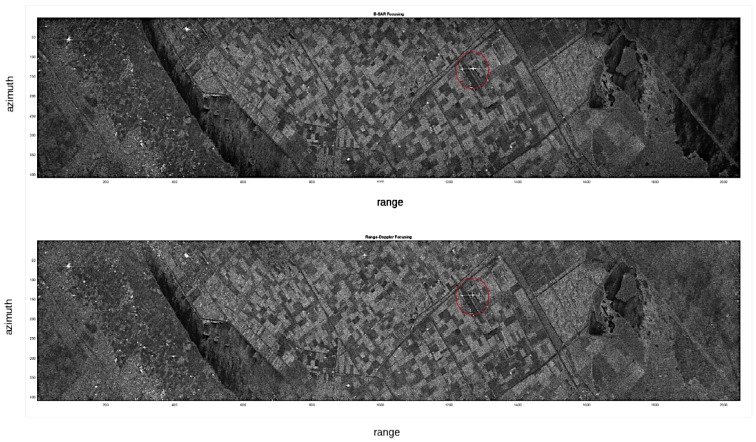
Sample of focused image with the proposed algorithm (upper) compared with the standard focusing obtained via Range Doppler algorithm (lower). ERS 1—Matera. The red circle indicates the point scatterer.
